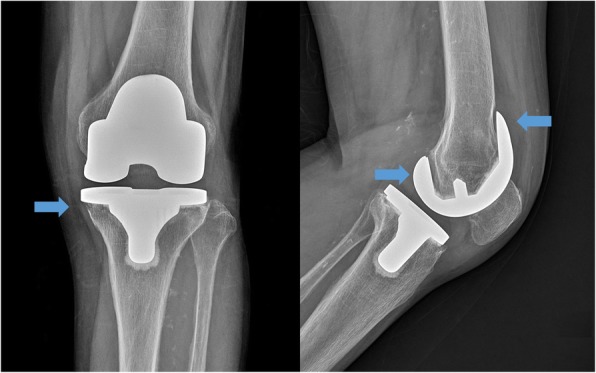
Plain radiograph from a 76-year-old female 3 years after primary total knee arthroplasty. Blue arrows indicate bone resorption around the femoral prosthesis and medial condyle of tibia
